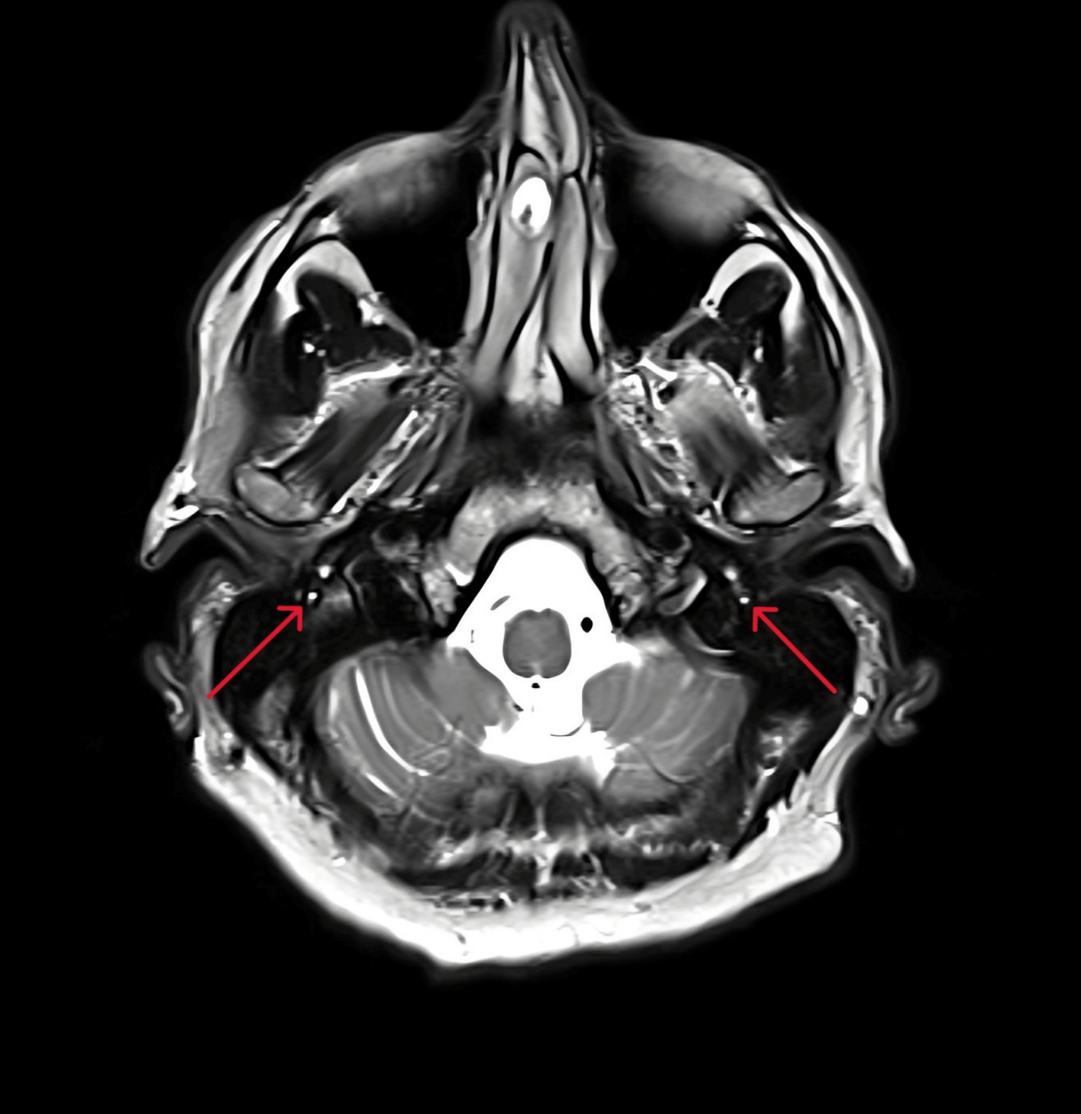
Contrast-enhanced MRI scan displaying abnormally high T2 signal of bilateral facial nerves, indicated by the red arrows.
